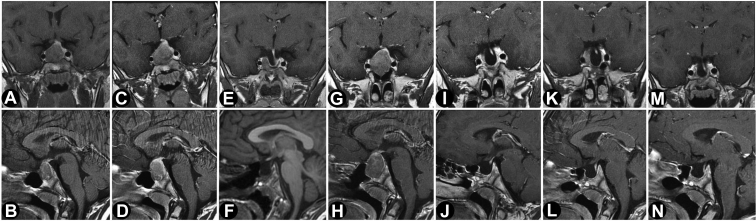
Sequential magnetic resonance imaging over the clinical course of the patient between 2018 and 2020. Contrast-enhanced T1-weighted sequences in coronal (*A, C, E, G, I, K, M*) and sagittal (*B, D, F, H, J, L, N*) planes were chosen and corrected for gray scale and magnification. The timing of the scans were as follows: at clinical presentation (*A, B*); before the first operation (lanreotide was switched to pasireotide; after this, magnetic resonance imaging was performed because of tumor growth, and surgery was performed because of visual field defects due to chiasmic compression) (*C, D*); postoperatively after the first operation (*E, F*); before the second operation (*G, H*); postoperatively after the second operation (1 month after intiating pegvisomant treatment) (*I, J*); before the third operation (*K, L*); and postoperatively after the third operation (*M, N*). There was no inhibition of tumor growth after the use of somatostatin analogs in terms of tumor size and extent. Correspondingly, the growth hormone secretion was normalized after the respective tumor resections. The tumor was medial to the intracavernous intercarotid line (Knosp status grade II); however, on direct vision during the last surgery, there was invasion of the cavernous sinus wall.
